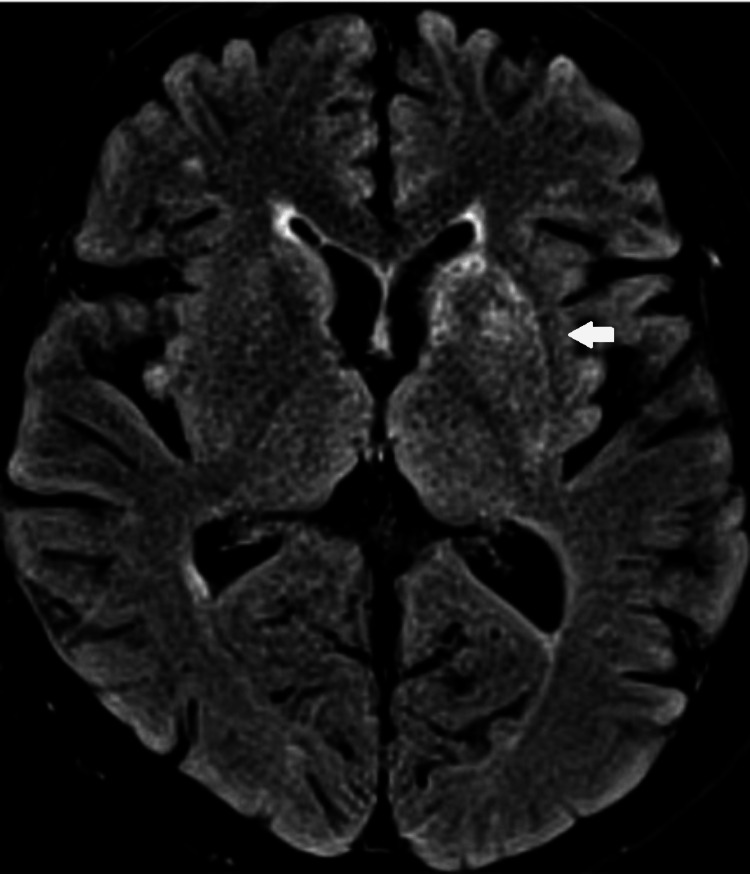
MRI stroke protocol - Reduced focal fluid attenuated inversion recovery (FLAIR) hyperintensity at the left lentiform nucleus
